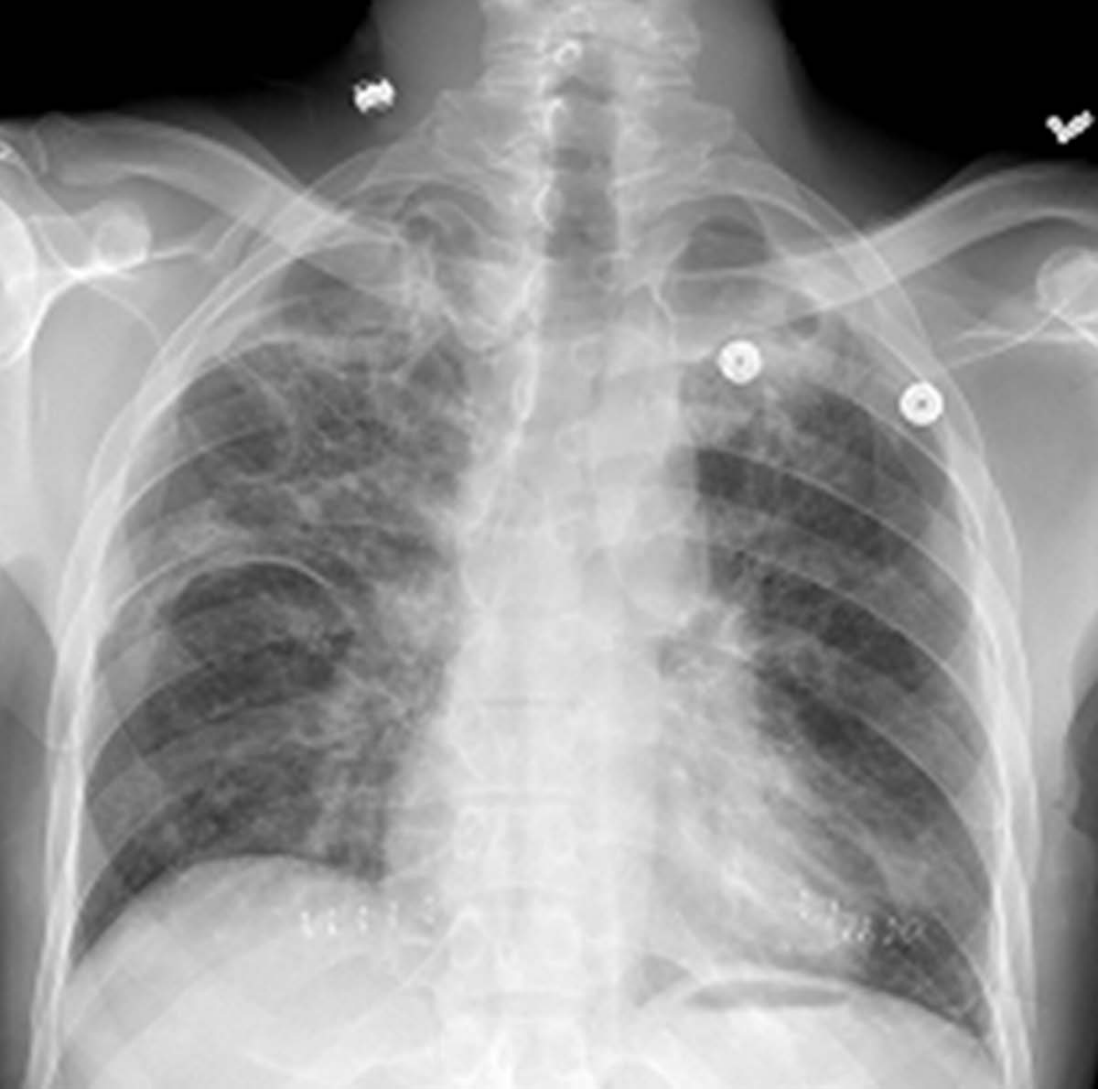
Chest radiograph at discharge showing improvement in infiltrates.
